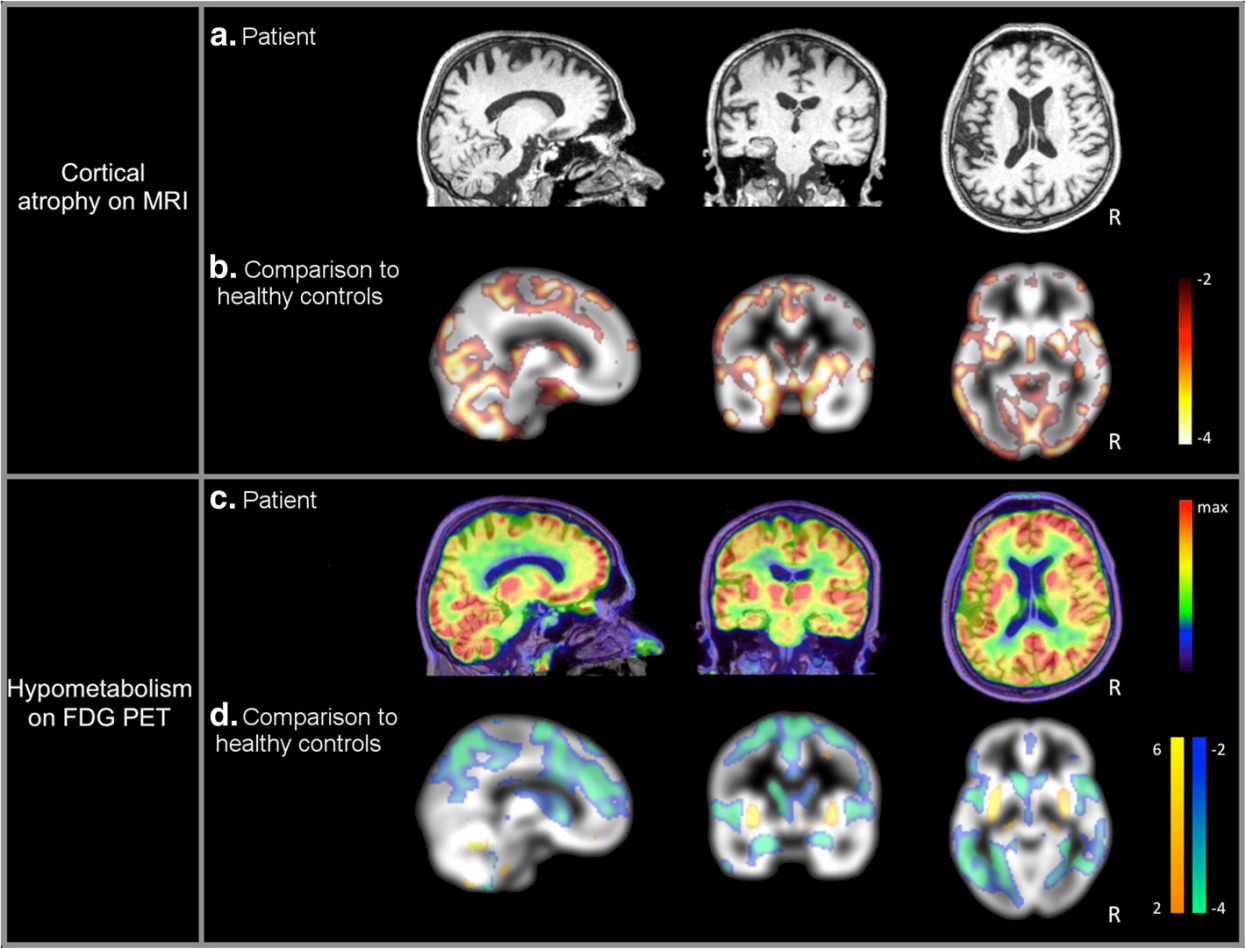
MRI and ^18^F-FDG-PET imaging. Patient’s MRI T1 sequence at M2 (a) and ^18^F-FDG–PETscan at M43 (c). Comparison between the patient and (b) age-matched healthy controls (*n* = 30) for grey matter density, (d) age-matched healthy controls (*n* = 23) for ^18^F-FDG uptake. Patient’s cortical atrophy below −2 standard deviations is displayed with a white to dark red scale. Patient’s hypometabolism below −2 standard deviations is displayed with a light green to dark blue scale while hypermetabolism is displayed with an orange to yellow scale. R = Right side
